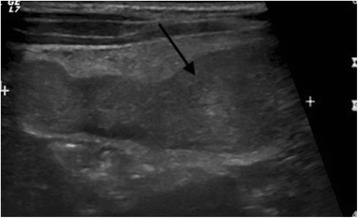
Ultrasound shows a hypoechoic fusiform mass (*arrow*)
